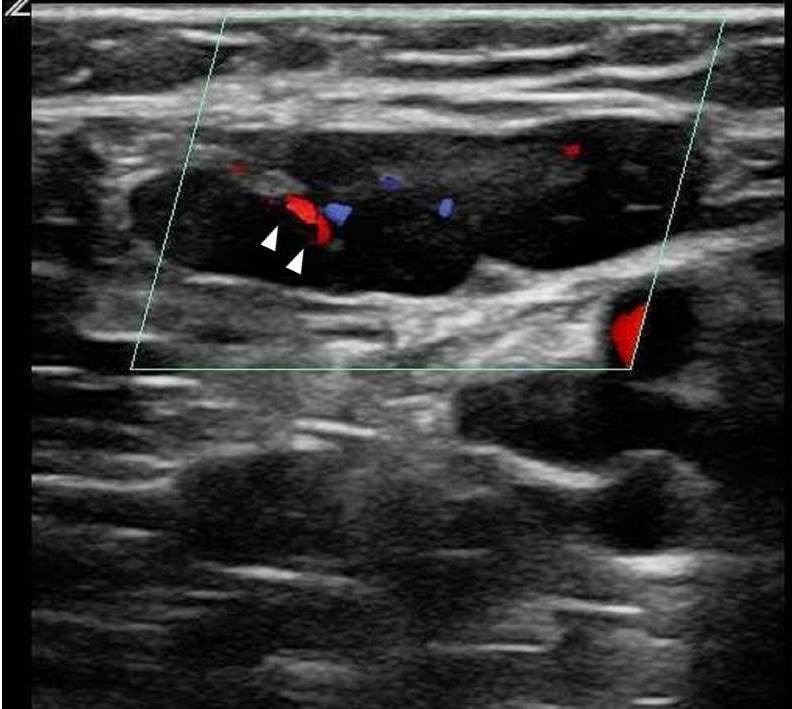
Enlarged inguinal lymph node (arrows) demonstrating flow in the hilar region with Color Doppler (arrowheads).
